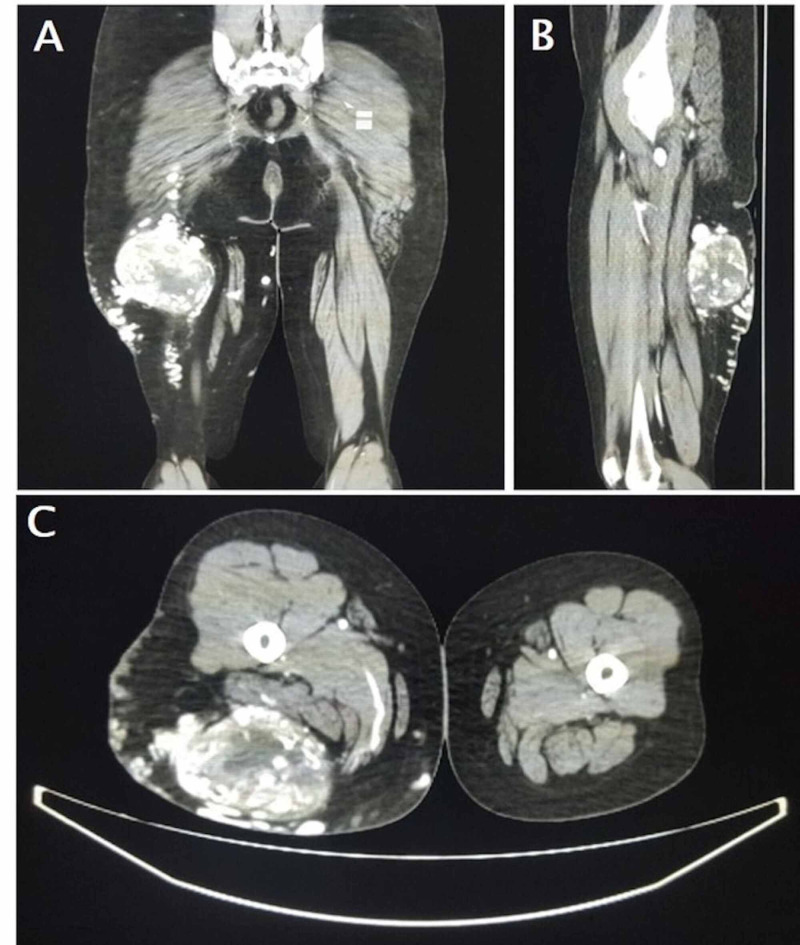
The coronal, axial, and sagittal CT scan images demonstrating the soft tissue tumor in the deep subcutaneous plane of upper thigh with no sign of bony erosion.
